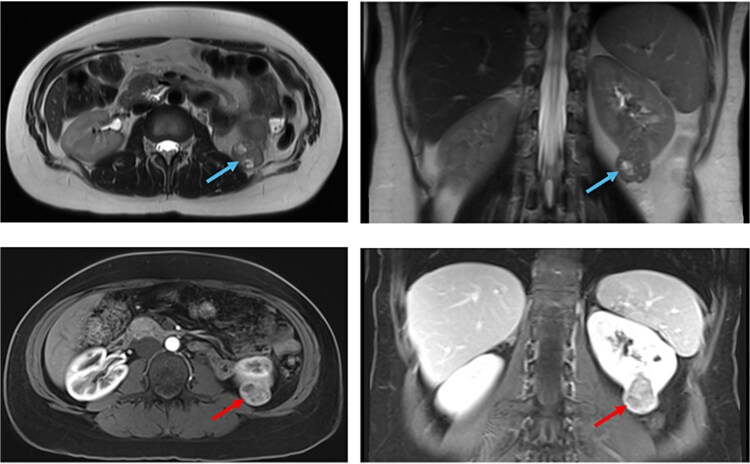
MRI examination of the abdomen showed a partially exophytic left renal mass on T2 sequence (blue arrows), and on contrasted T1 sequence (red arrows).
